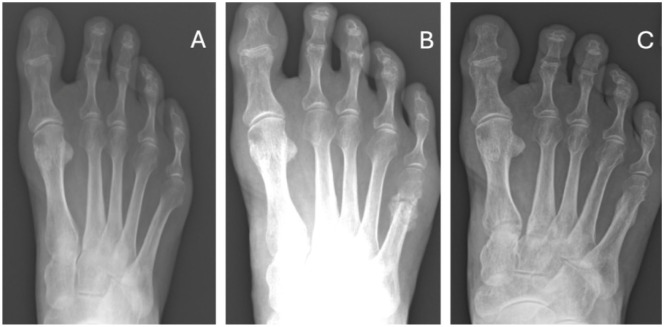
(A) Pre-operative radiograph showing lateral deviation of the fifth metatarsal head. (B) 12-week postoperative radiograph demonstrating osteotomy site healing with significant hypertrophic callus formation. (C) 12-month radiograph showing complete remodeling and resolution of callus at the osteotomy site.
